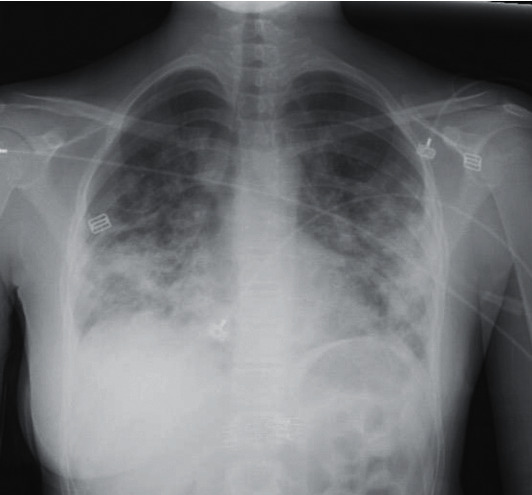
Admission chest radiograph demonstrates bilateral ill-defined opacities with mid and lower lung predominance.
